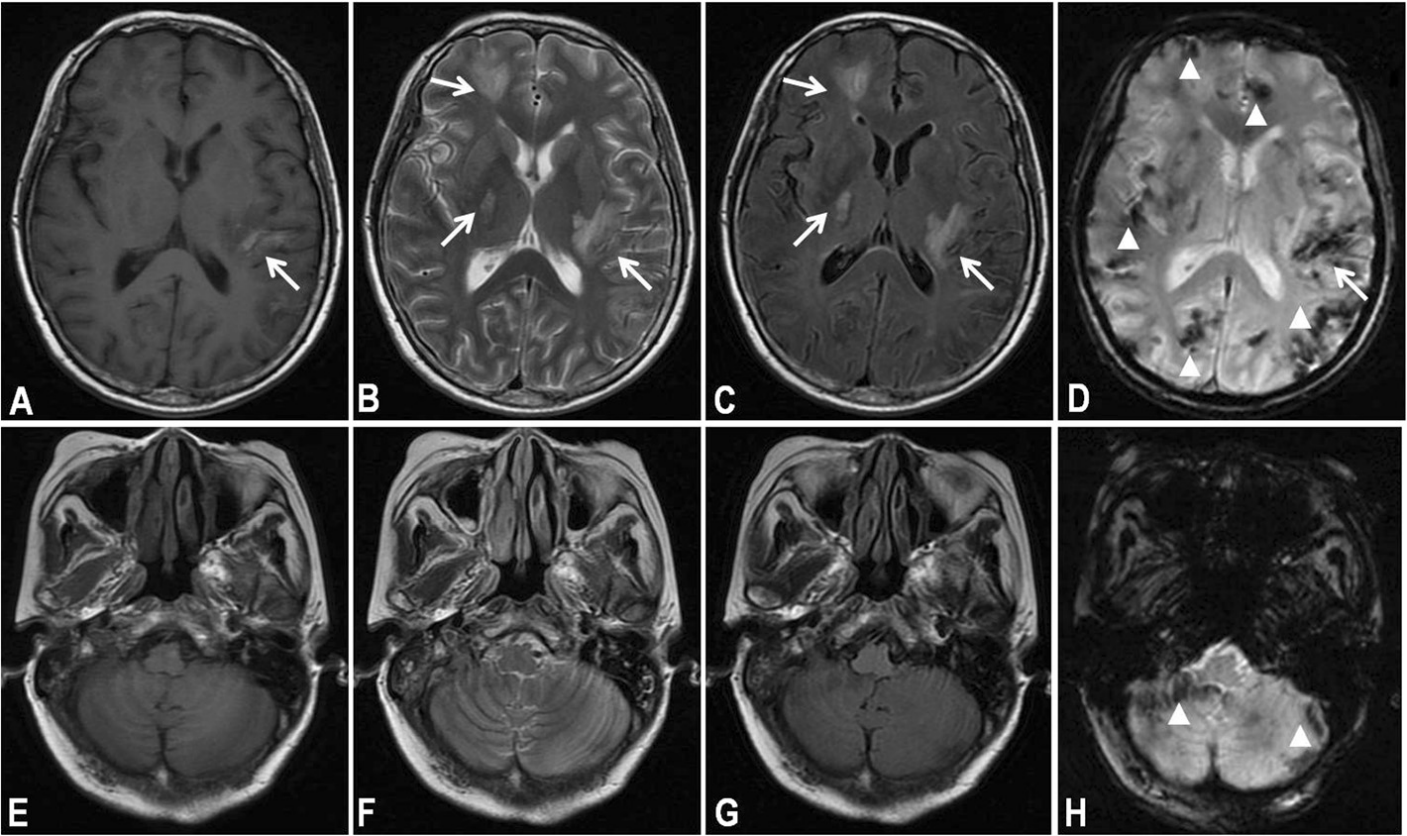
Axial brain magnetic resonance imaging including supratentorial and infratentorial regions after admission. (A) & (E) T1-weighted imaging demonstrated a hypointense area with focal hyperintensity in the left temporal lobe (arrow), suggesting newly patchy hemorrhage within the lesion. (B) & (F) T2-weighted imaging demonstrated multiple hyperintense lesions only involving the supratentorial region (arrows). (C) & (G) Fluid attenuated inversion recovery (FLAIR) showed multiple hyperintense lesions only involving the supratentorial region (arrows). (D) & (H) T2*-weighted MRI demonstrated diffuse hypointensities (arrowheads), indicating multifocal hemosiderin depositions in both supratentorial and infratentorial regions, which were not compatible with the hemorrhagic lesions visualized on T1-weighted images.
